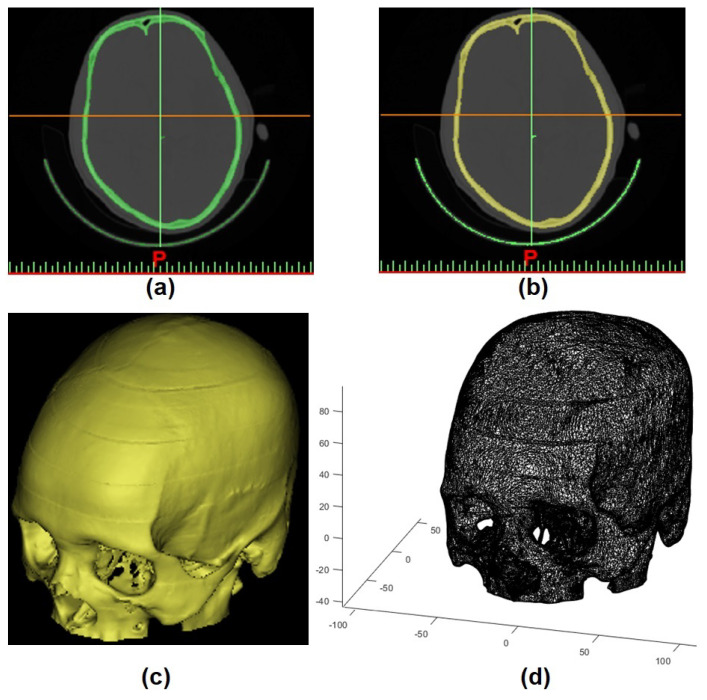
(a) thresholding applied in CT scan, (b) region growing, (c) 3D mesh model (STL model), and (d) STL model converted into matrix.
